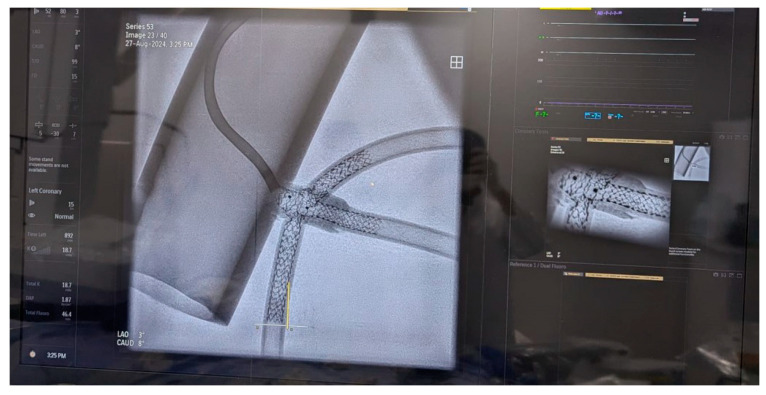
In vitro procedureunder X-rays.
